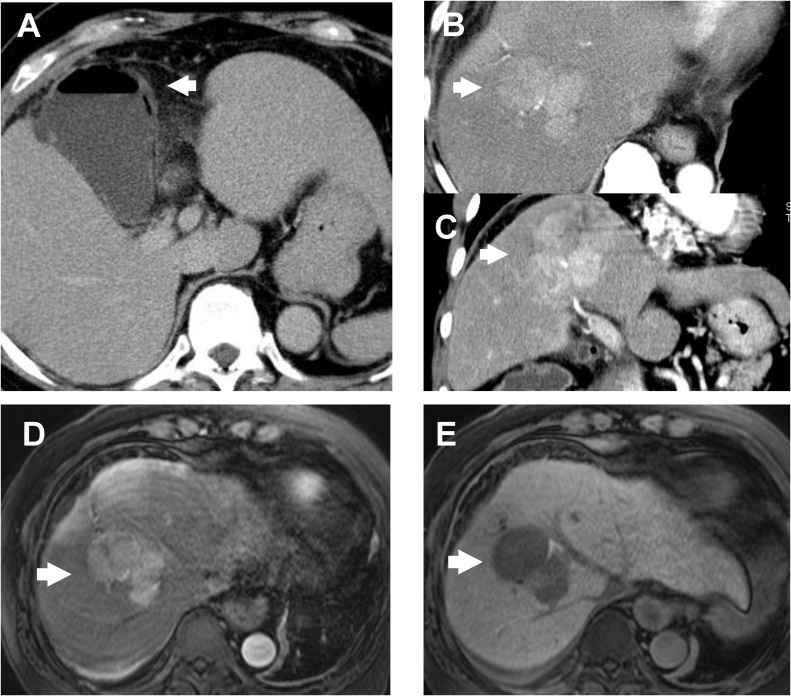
Abdominal computed tomography revealed wall thickness of the gallbladder with air density (A) and an 80-mm tumor with early enhancement in the segment 8 of the liver tumor (B–C, arrow). Gadoxetic acid-enhanced magnetic resonance imaging demonstrated a liver tumor, which had hyper vascularity in arterial phase (D) and washout in hepatocyte phase (E).
